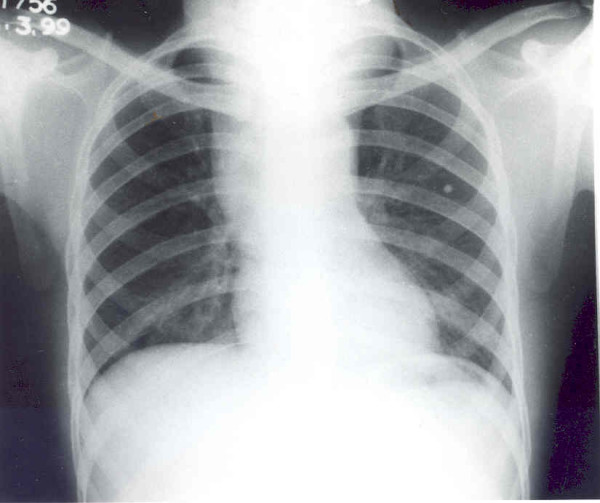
Chest x-ray (P-A view) showing widening of the mediastinum and a soft tissue swelling in the neck, continuous with the mediastinal shadow.
